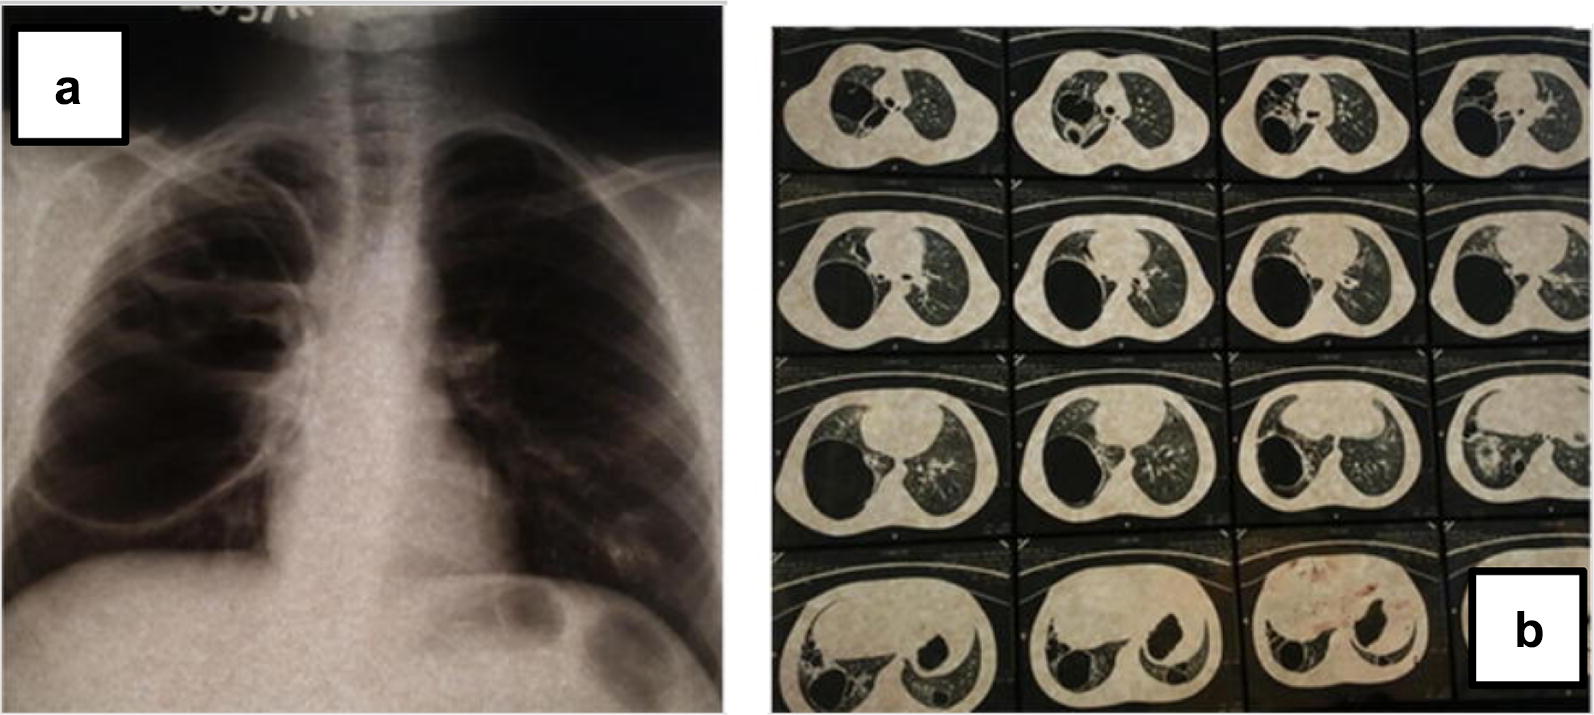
Radiological manifestations of patient 1. a Chest X ray and b High-resolution computed tomography (HRCT) of chest
